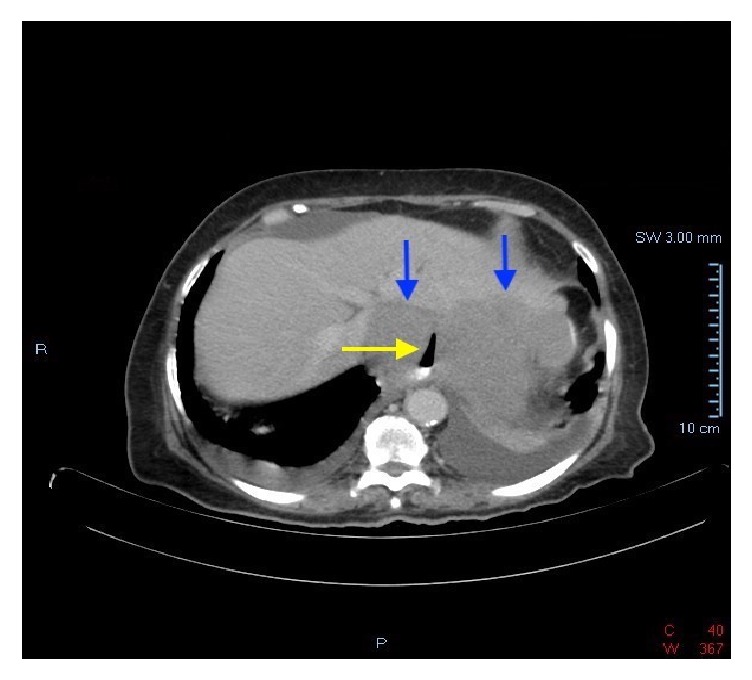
CT scan (axial view) showing a 12 x 12 soft tissue mass in the gastrohepatic omentum (blue arrows) compressing the distal esophagus (yellow arrow) and gastric fundus.
